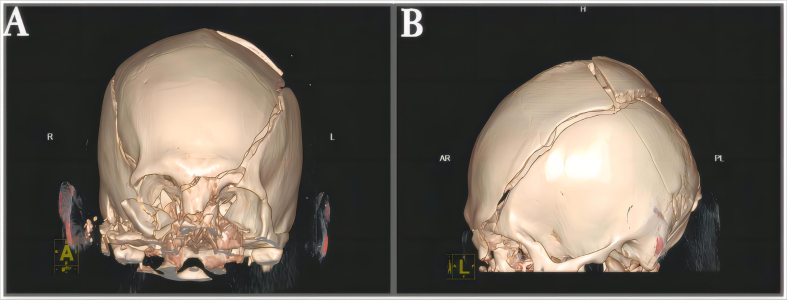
(A and B)The three-dimensional reconstructed image of the first head CT scan upon the patient's admission.
